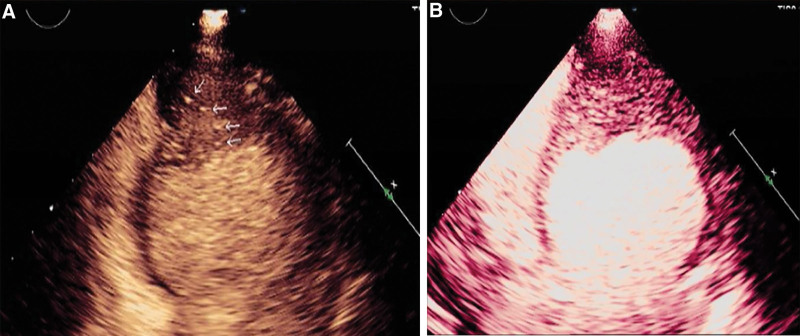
Contrast echocardiography showing apical calcification (A, white arrow) and the “apple” sign of the morphology of the left ventricular chamber with contrast agent filling (B).
